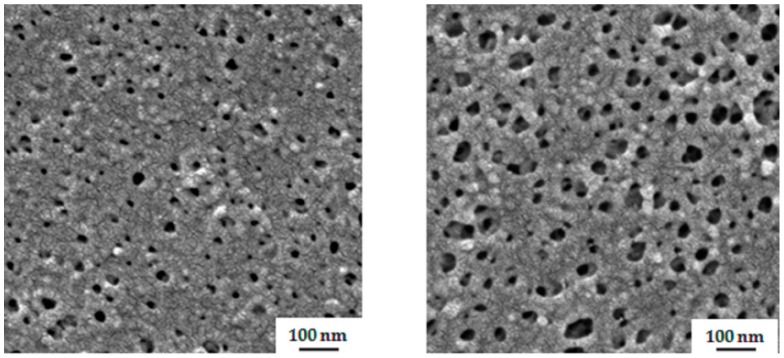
SEM images of the top surface of cast membranes from 24 wt % PS_76_-PSMA_24_^200^ from (left) THF/DMF 50/50 wt %, (right) THF/DMF 40/60 wt %. The time of evaporation before immersion into precipitant was 10 s.
